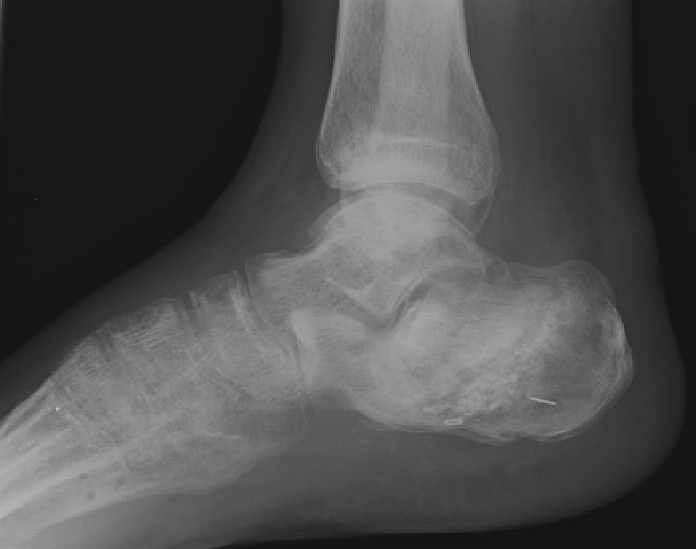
Five months post-injury lateral ankle x-ray.
